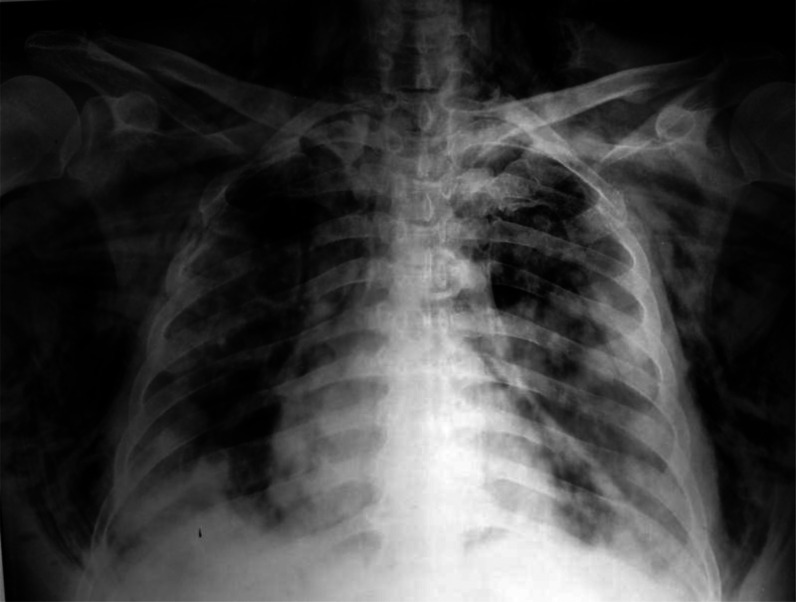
Chest radiograph showing bilateral peripheral opacities in the lung fields, bilateral pneumothorax, and subcutaneous emphysema.
